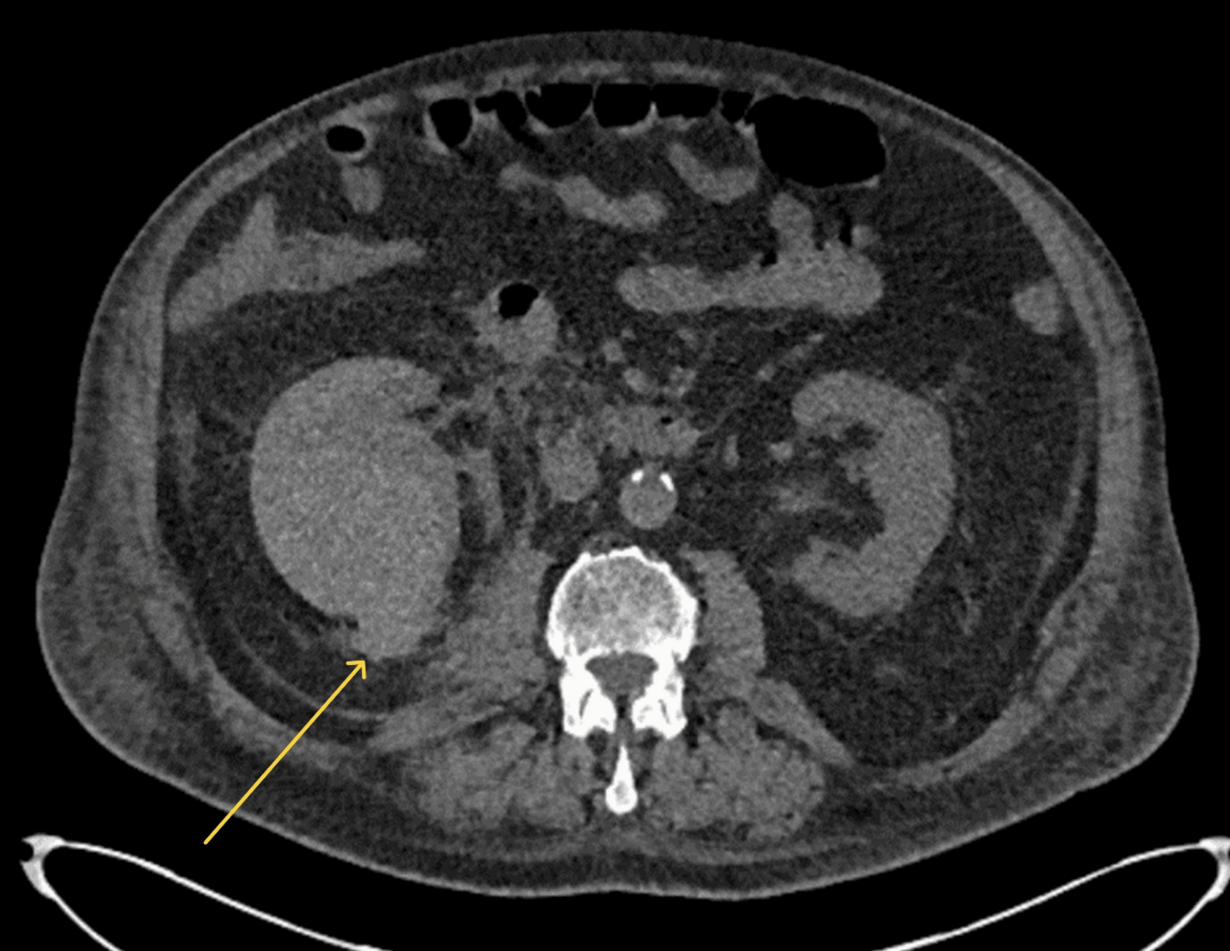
CT abdomen (axial view) in the portal venous phase The right kidney is oedematous with hyperdense peripheral content (arrow), suggesting a subcapsular haematoma. The hyperdensity tracks posteriorly to the perinephric space, indicating cortical disruption. CT, computed tomography
